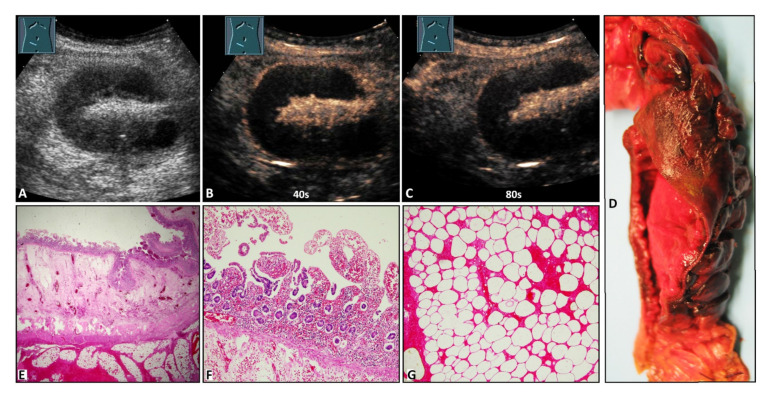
A 42-year-old male patient with acute peritoneal pain in the right lower abdomen and a palpable subcutaneously located tumor in the form of a Spieghel’s hernia. (A) B-mode ultrasound shows a standing loop of small bowel. (B,C) Contrast-enhanced ultrasound shows absent enhancement of the bowel wall after 40 s and 80 s. (D) Macroscopic evaluation shows a color change to deep red in the mucosa in an 8 cm long segment. (E) Cross section of the small intestine with luminal mucosa, edematous submucosa, muscularis, and hemorrhagic submucosa (from top to bottom). The mucosa shows incomplete ischemic necrosis with vital crypt epithelium (1.25× magnification). (F) Small intestinal mucosa with incomplete ischemic necrosis with vital crypt epithelium (10× magnification). (G) Necrotic mesenteric fat tissue with missing nuclei and hemorrhages (10× magnification).
